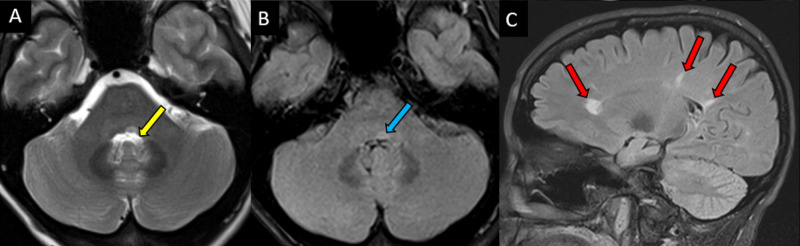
A 52-year-old male with a known history of multiple sclerosis presented with dizziness and weakness, but reported no extraocular muscle dysfunction. (A, B) Axial T2-weighted (top left) and FLAIR sequence (top right) demonstrating a small, focal demyelinating plaque in left dorsal pons (yellow and blue arrows). (C) Sagittal FLAIR sequence demonstrating the characteristic periventricular appearance of whiter matter plaques. FLAIR: fluid-attenuated inversion recovery
